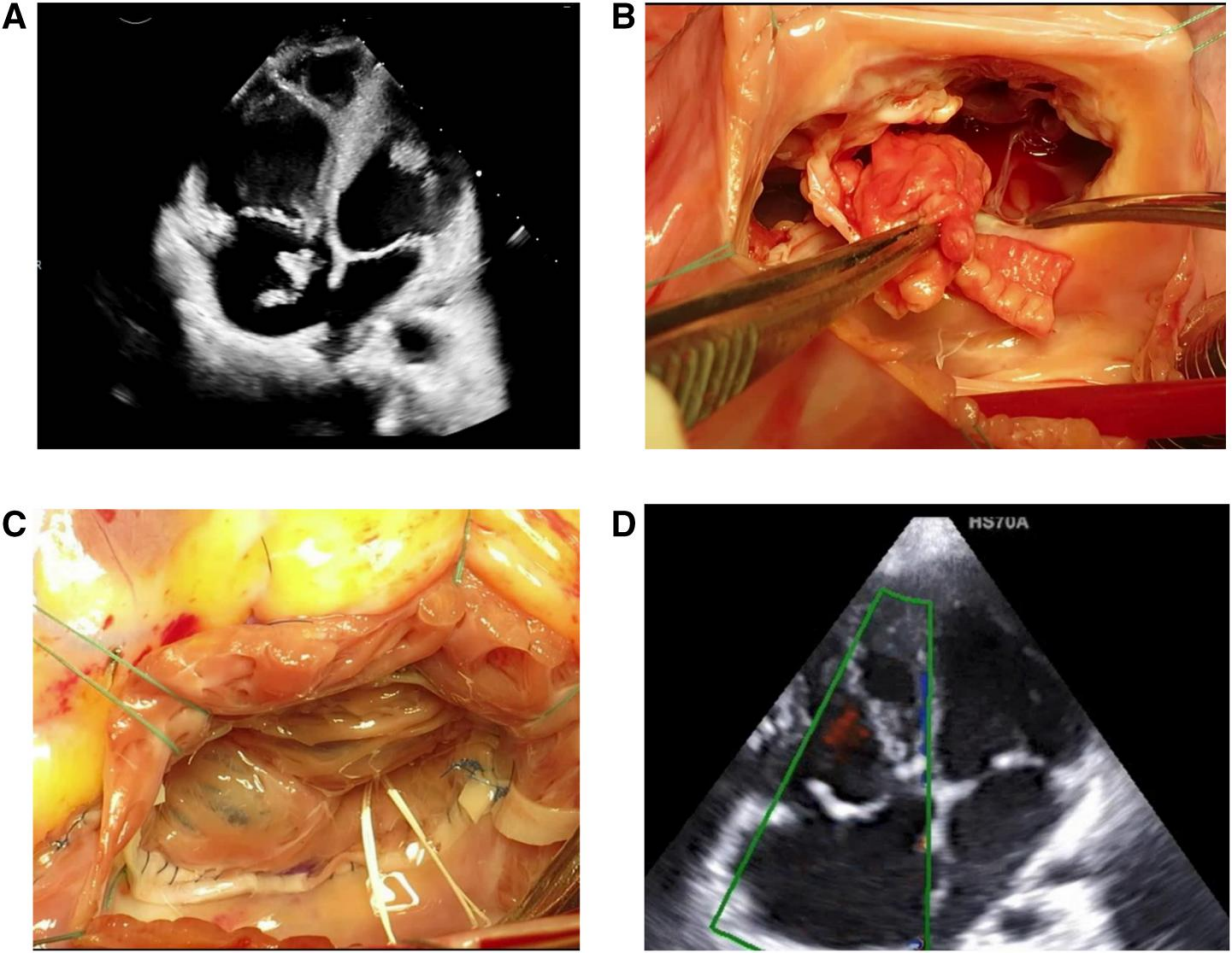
Right Atrial Appendage Valve Replacement for Tricuspid Valve Destruction Due to Infective Endocarditis. (A) Tricuspid valve destruction with large vegetation from infective endocarditis. (B) Multiple large vegetations leading to extensive leaflet destruction, making repair unfeasible. (C) Post-repair view showing well-coapted RAA valve with no regurgitation, using artificial chordae for positioning. (D) Reconstructed valve showing improved leaflet coaptation and satisfactory positioning, with no significant regurgitation on colour Doppler
